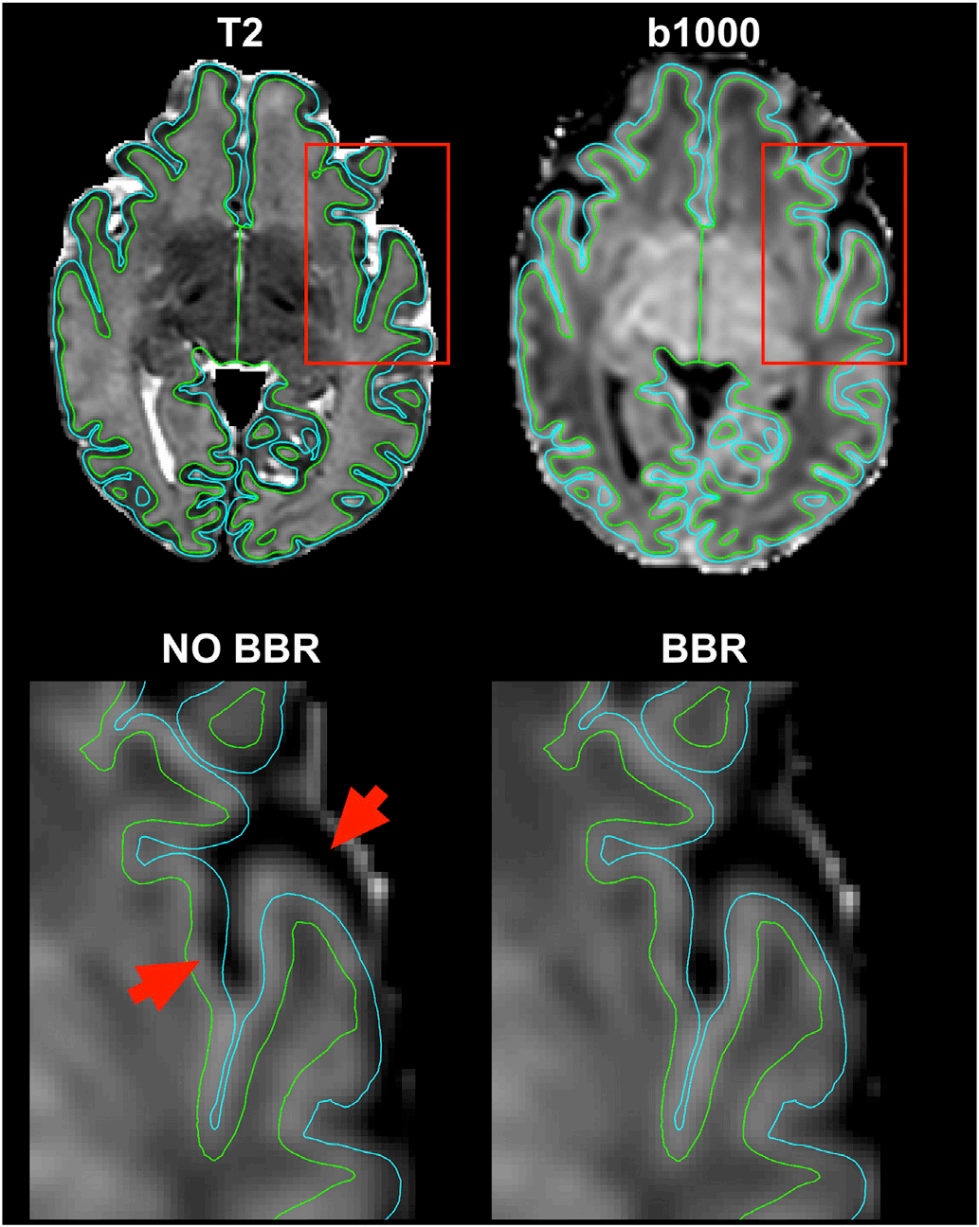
Registration accuracy using BBR. The fully pre-processed average attenuation profile volume for the b = 1000 s/mm^2^ shell is registered to the high resolution T2-weighted volume in subject's native space (top row). When using BBR the tissue boundaries are better aligned to those extracted from the high-resolution volume, as shown by the two insets (red box). Red arrows point to areas where standard volumetric registration resulted in residual misalignments in the left hemisphere (bottom row). White/grey matter (green) and grey/csf boundaries (light blue) are overlaid on top of the attenuation profile transformed to the T2 anatomical space.
